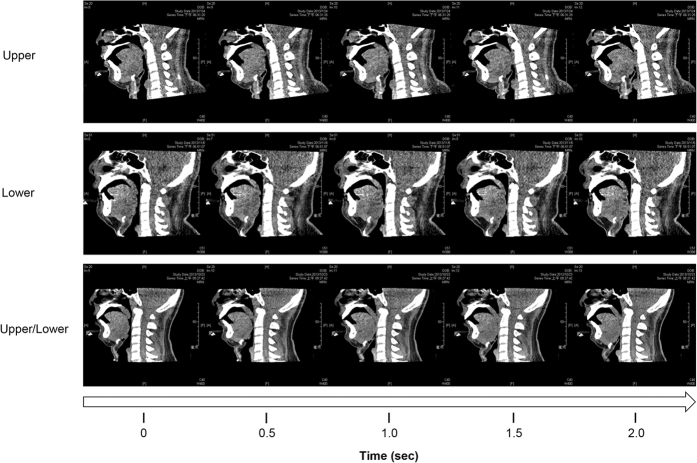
Types of tongue obstruction. Upper, lower and upper/lower types of tongue obstruction were noted in drug-induced sleep computed tomography.
